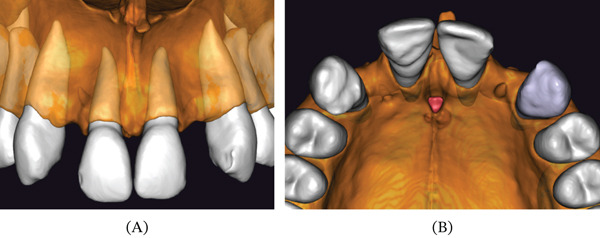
(A, B) 3D model from CBCT images before implant insertion.
